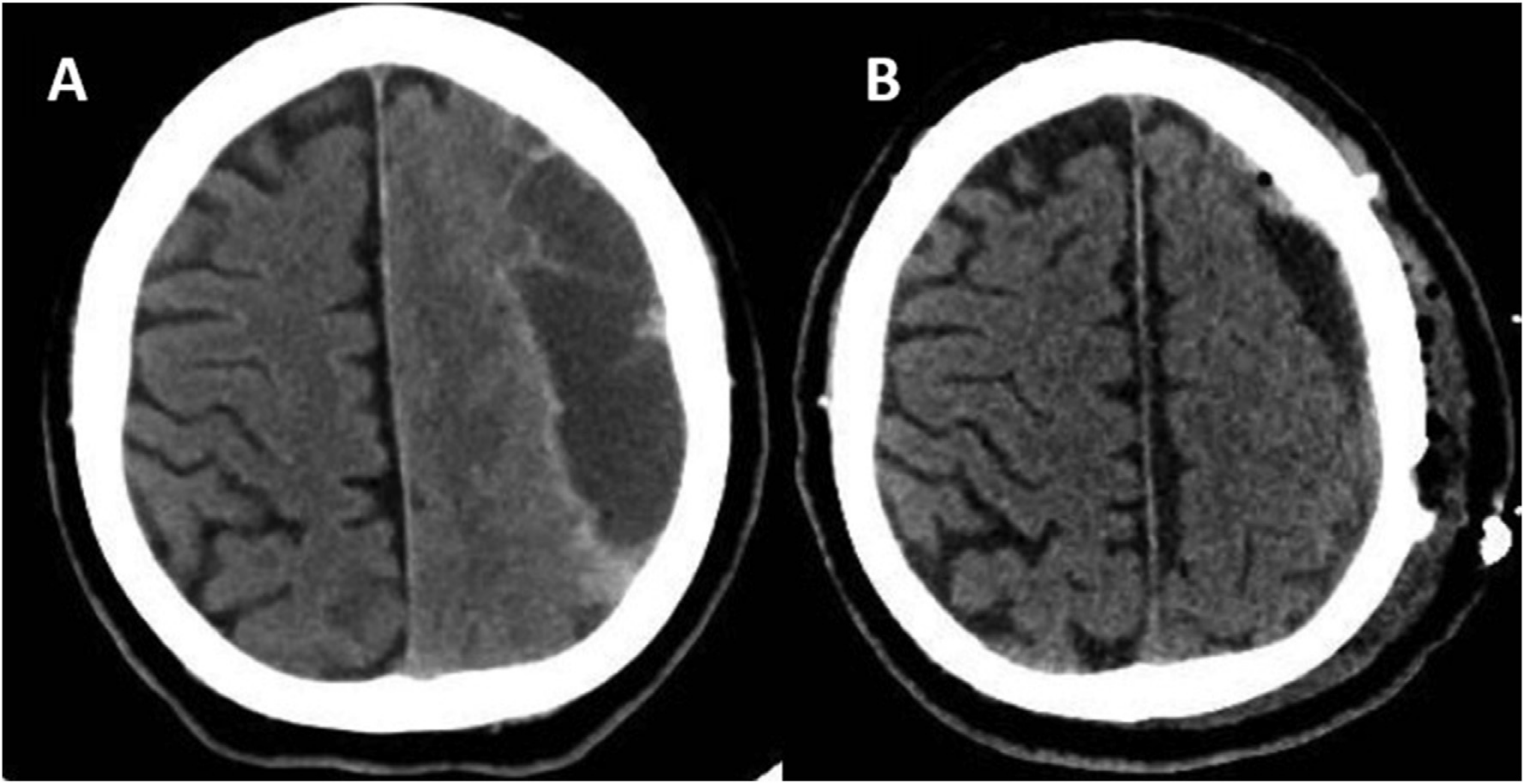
Example of a brain computed tomography scan of a 75-year-old male before and after successful burr hole drainage.
